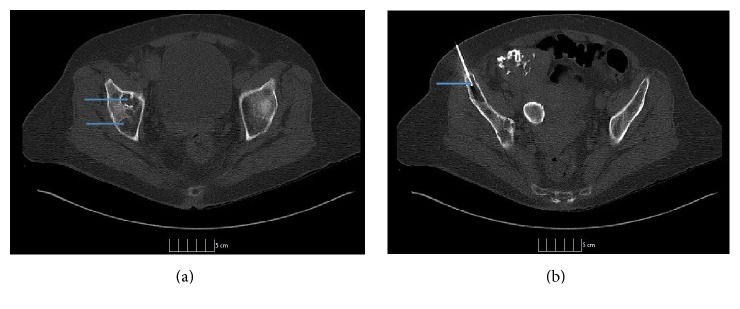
CT imaging of the pelvis showing cystic osteolytic (exceptional) bony lesion with a sclerotic rim within the (a) right acetabulum and (b) right iliac crest with FNAC needle.
